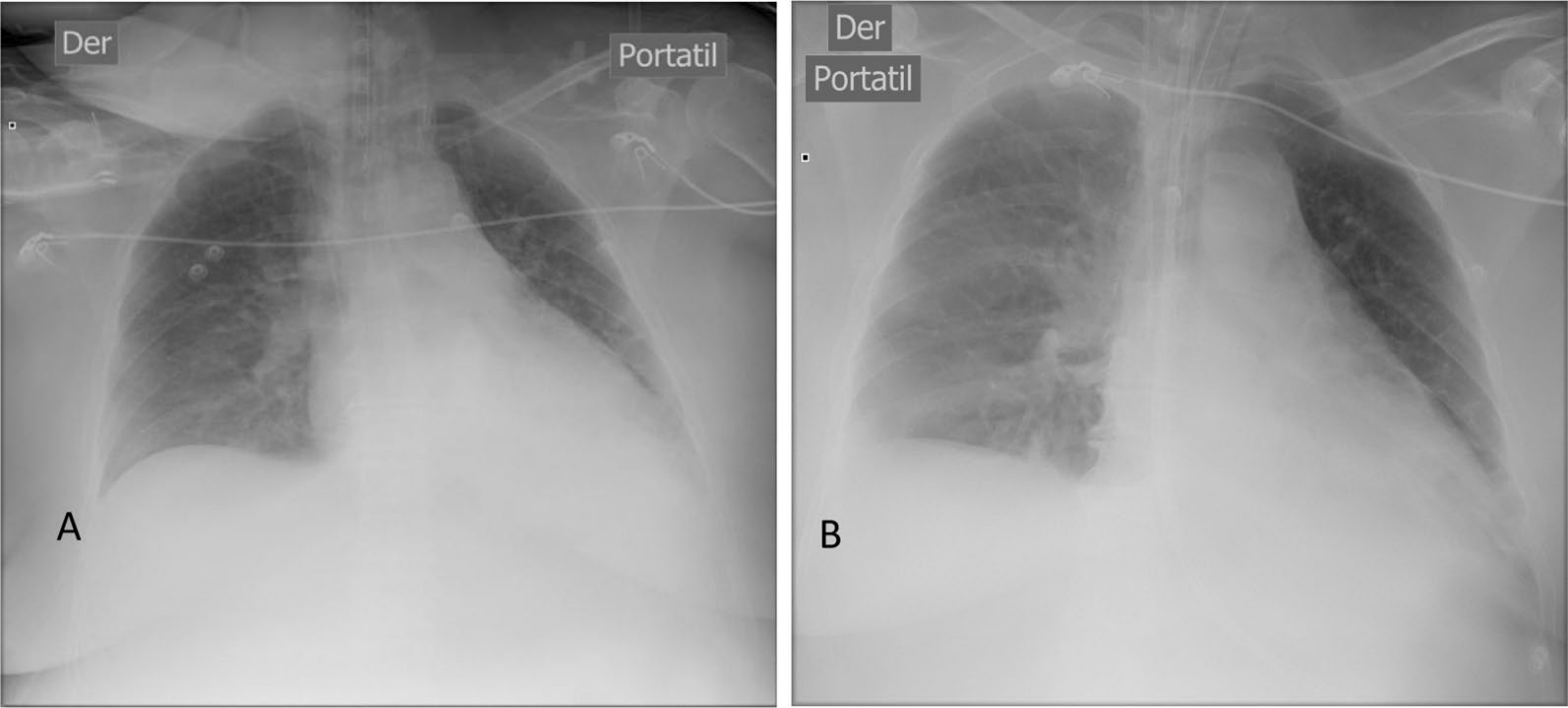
A Thoracic X-ray on first day in intensive care unit / B Thoracic X-ray on third day in intensive care unit
